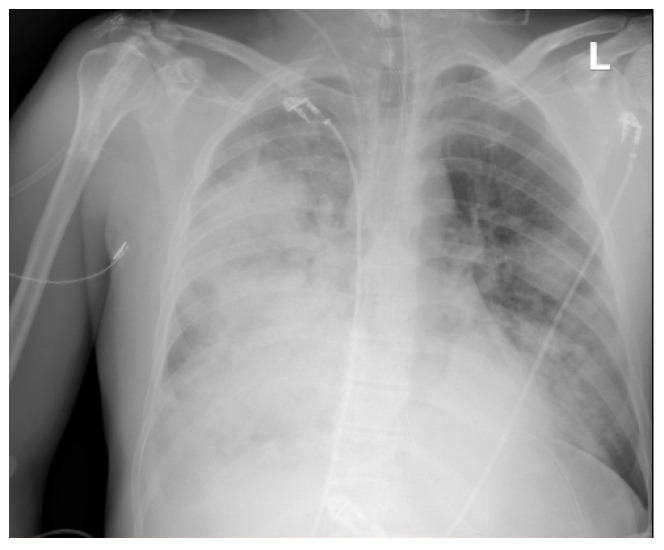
Chest X-ray. Pneumonia caused by *S. pyogenes*. Patient on veno-venous extracorporeal membrane oxygenation (VV-ECMO).
